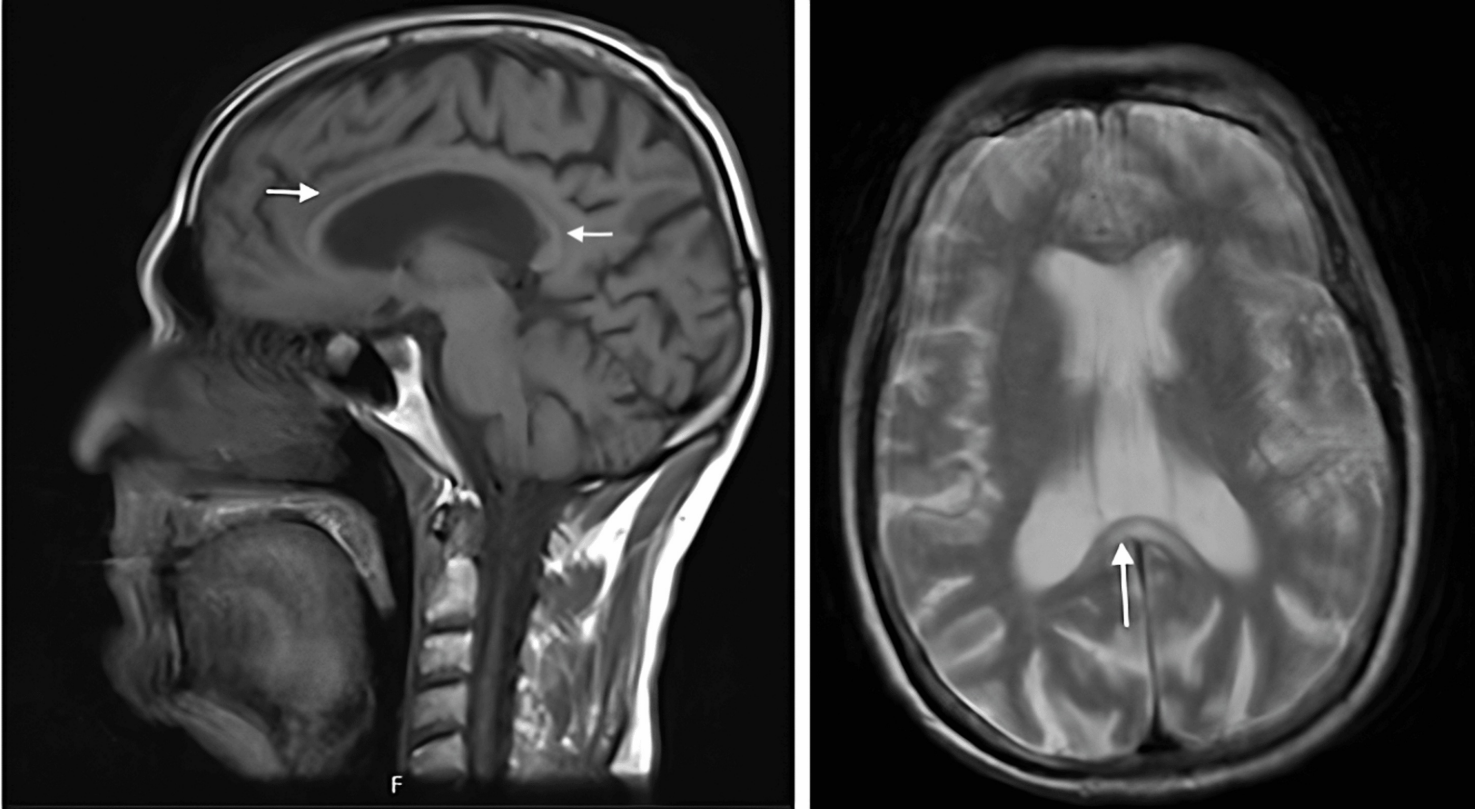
Brain MRI demonstrating marked atrophy of the corpus callosum, particularly involving the body and splenium, with hypointensity on T1 and hyperintensity on T2-weighted images.
